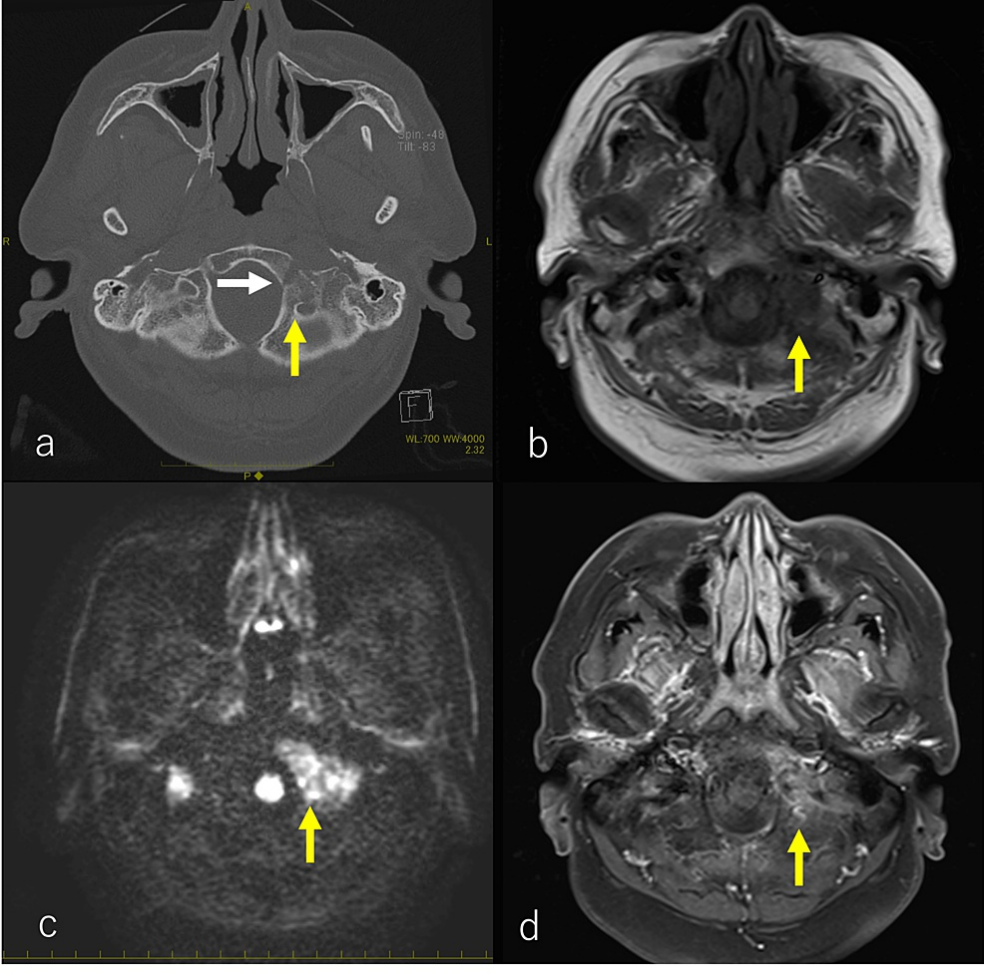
Pretreatment CT and MRI findings. (a) CT bone scan revealed osteolytic bone metastasis extending from the left side of the oblique plateau to the occipital bone (yellow arrow) with invasion into the sublingual neural tube (white arrow). (b) MRI scan showed a low-signal area in the region identified on CT in the T1-weighted image (yellow arrow) and (c) a high signal in the same area in the diffusion-weighted image (yellow arrow). (d) Gadolinium contrast-enhanced CT also demonstrated a contrast enhancement effect (yellow arrow).
